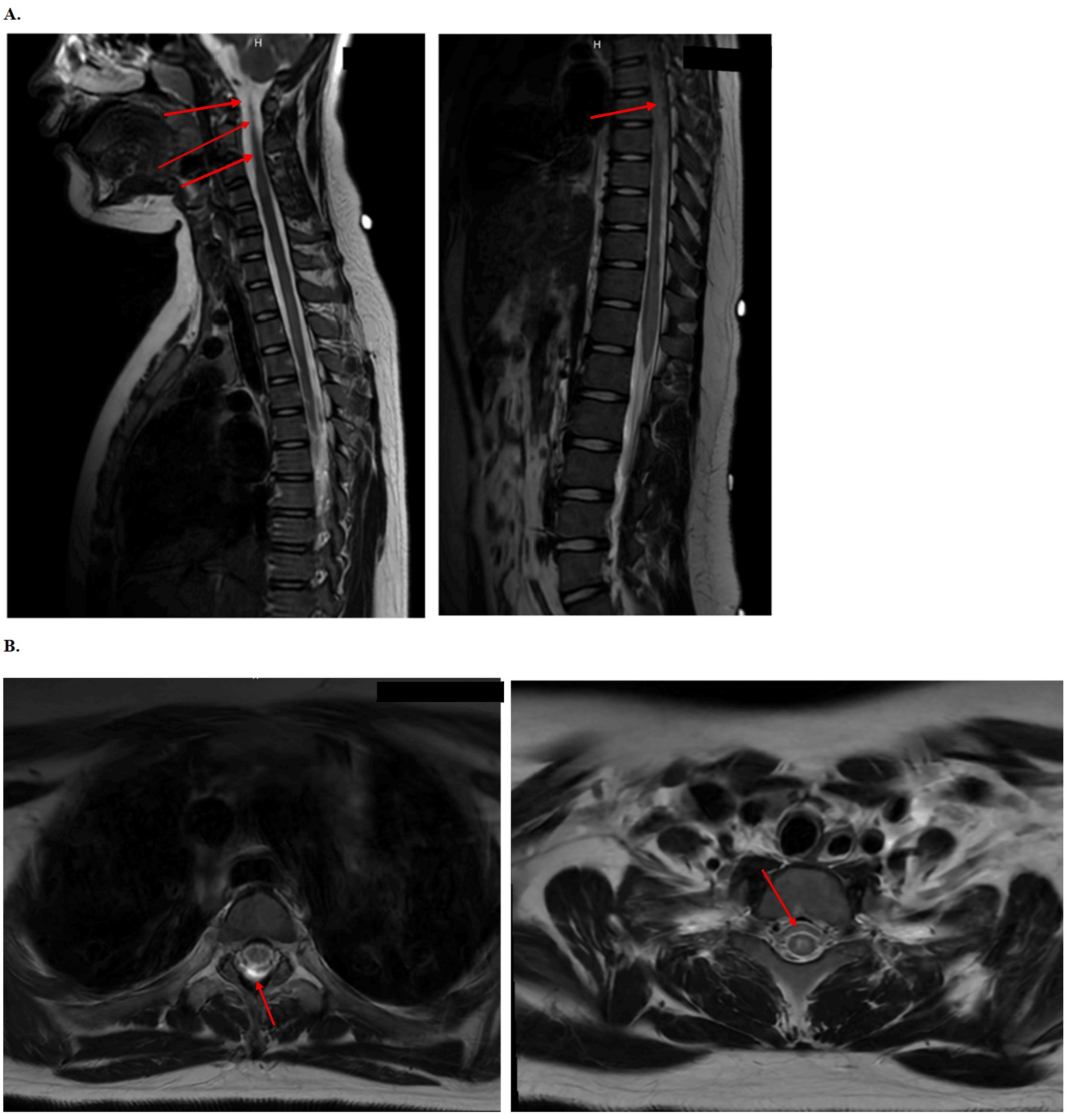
Sagittal and axial T2-weighted MRI images of the cervical, thoracic, and lumbar spine demonstrating multifocal lesions A. Sagittal T2-weighted MRI images of the cervical (left) and thoracic/lumbar (right) spine. The cervical spine image (left) demonstrates multifocal, intermittent, patchy areas of increased T2 signal intensity extending from the cervical spinal cord at C2 (uppermost arrow), as well as additional areas of involvement at C5-C6 and the thoracic spine. The thoracic/lumbar spine image (right) highlights the extent of T2 hyperintensity extending down to the conus medullaris, terminating at the superior endplate of L1. B. Axial T2-weighted MRI images at the level of C2 (left) and the thoracic spine (right). These axial images provide a detailed view of the topography and distribution of the lesions within the spinal cord, particularly at the C2 level (left image, arrow), where the lesion is characterized by increased signal intensity that is better visualized in this plane. The axial thoracic image (right) shows the corresponding transverse extent of the thoracic lesion.
